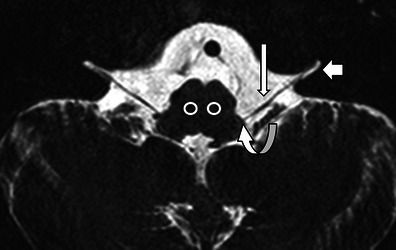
The vagal nerves in the posterior fossa.*Axial heavily T2-weighted thin-slice MR image through the brainstem with the position of the nucleus ambiguus (*white circles*), the exit of the vagal nerves from the olivary sulcus (*curved arrow*), the cisternal segment of the vagal nerves (*long arrow*), and the pars nervosa of the jugular foramen (*short arrow*)
